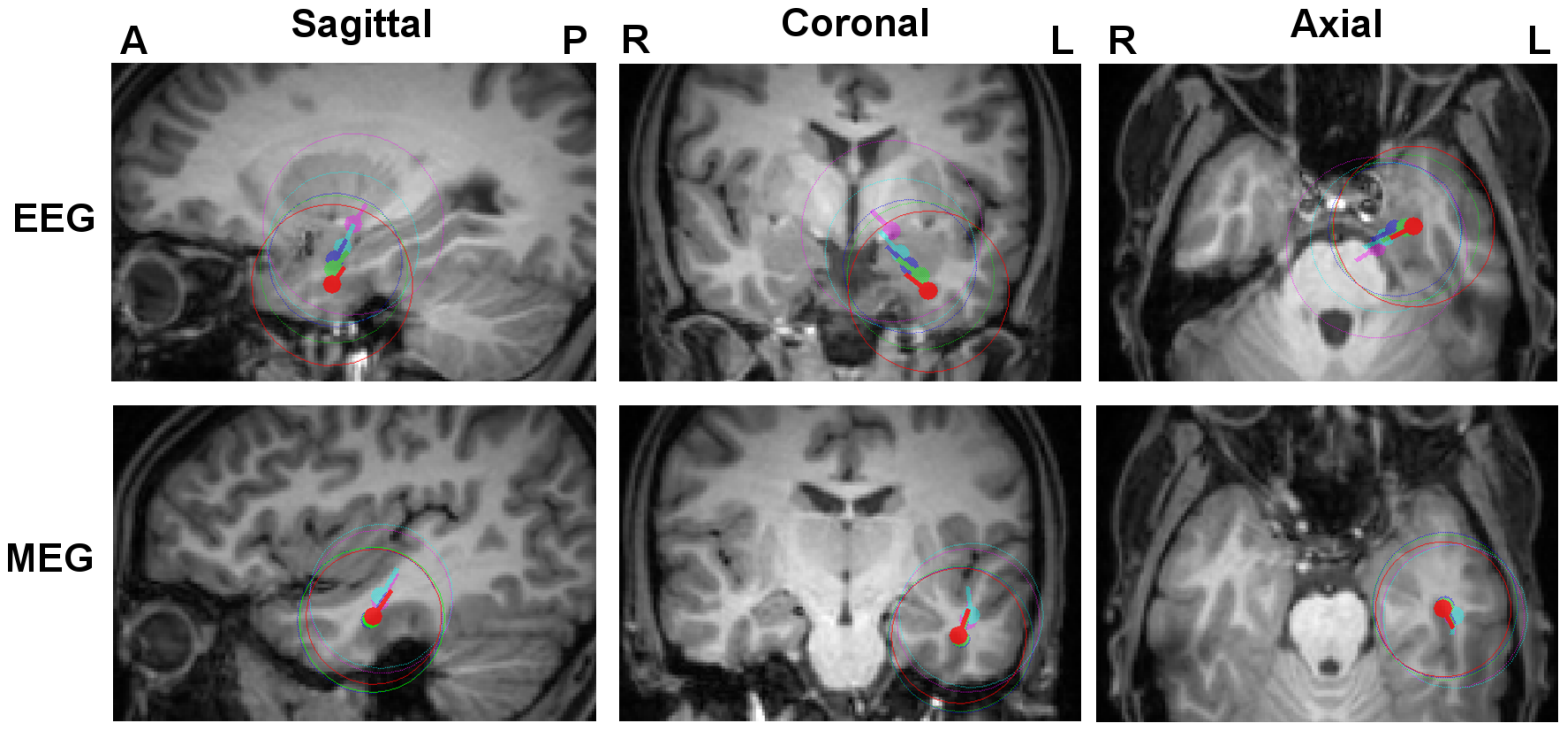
Influence of skull conductivity on EEG and MEG localizations. FT9 centroids and spread spheres plotted on T1w-MRI for head models *6C_Cal* (red), *6C_41* (green), *6C_70* (blue), *6C_132* (cyan) and *6C_330* (magenta). The centroid locations of *6C_Cal* were used for the selection of MRI slices and all results were projected on these slices.
